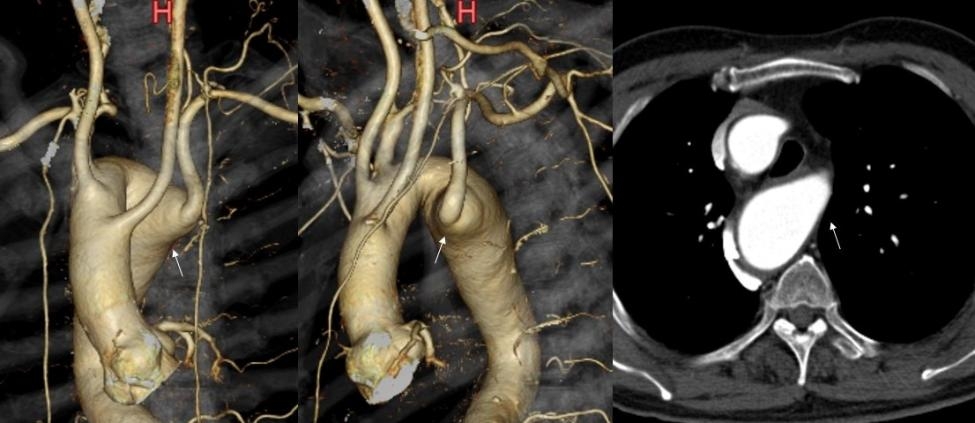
Preoperative computed tomographic angiography revealed a right aortic arch, Kommerell’s diverticulum (arrow), and an aberrant left subclavian artery
